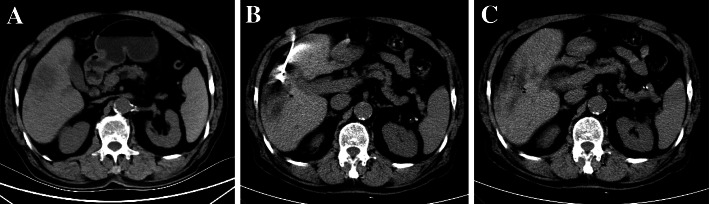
CT imaging before ablation therapy a and after placement of an MWA probe b. Postinterventional CT imaging after removal of the MWA probe shows a non-enhancing ablation zone c. The path of the former probe track can still be seen lateral to the ablation zone. In the center of the ablation zone the formation of bubbles after tissue ablation
